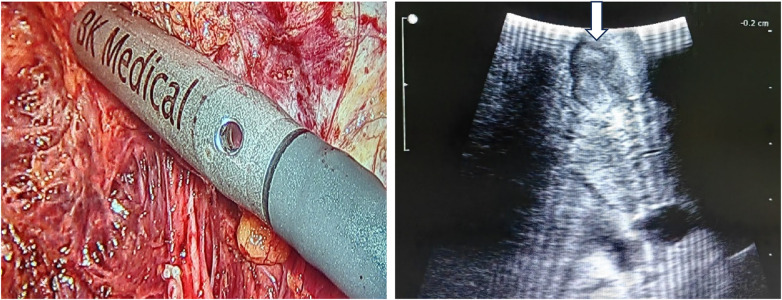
Intraoperative ultrasound delineating the tumor margin and guiding the resection line.
